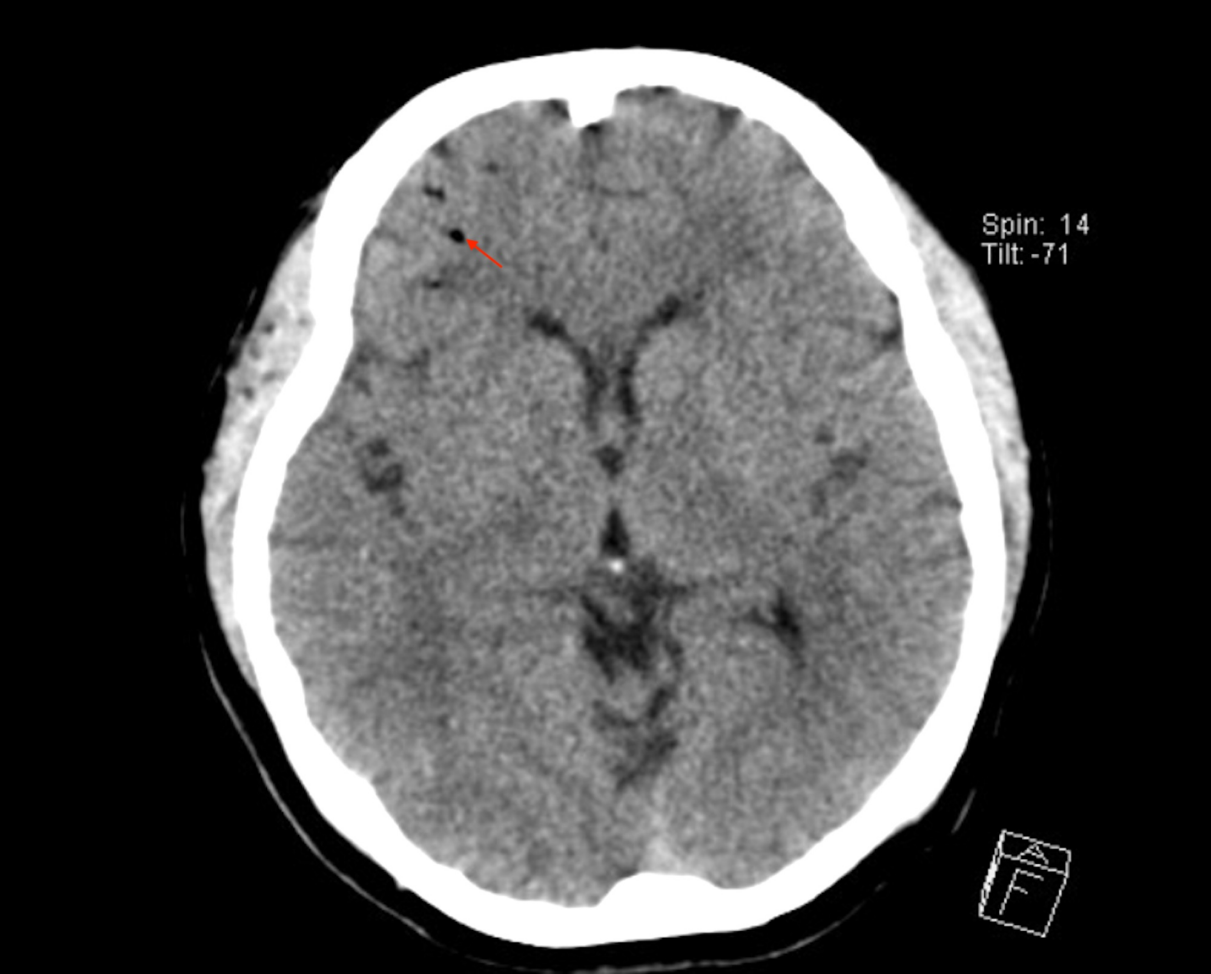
Computed tomography (CT) head. CT head showing multiple air emboli (arrow).
